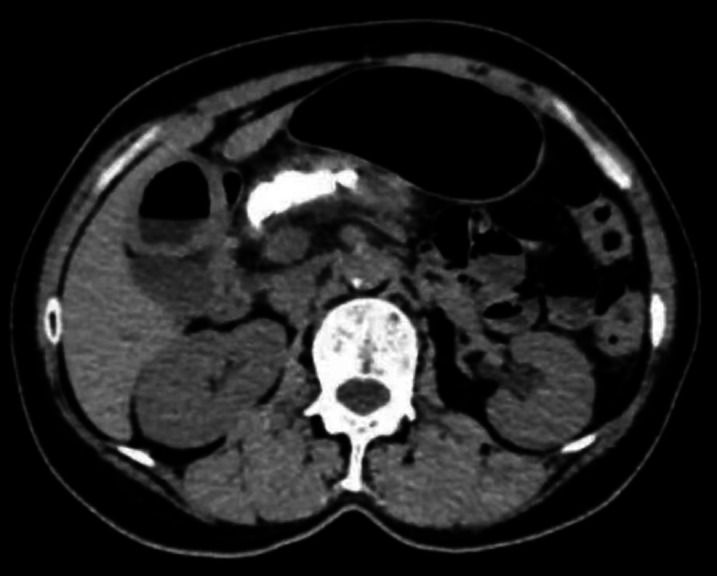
Computed tomography image showing chronic pancreatitis and pancreatic duct stones.
